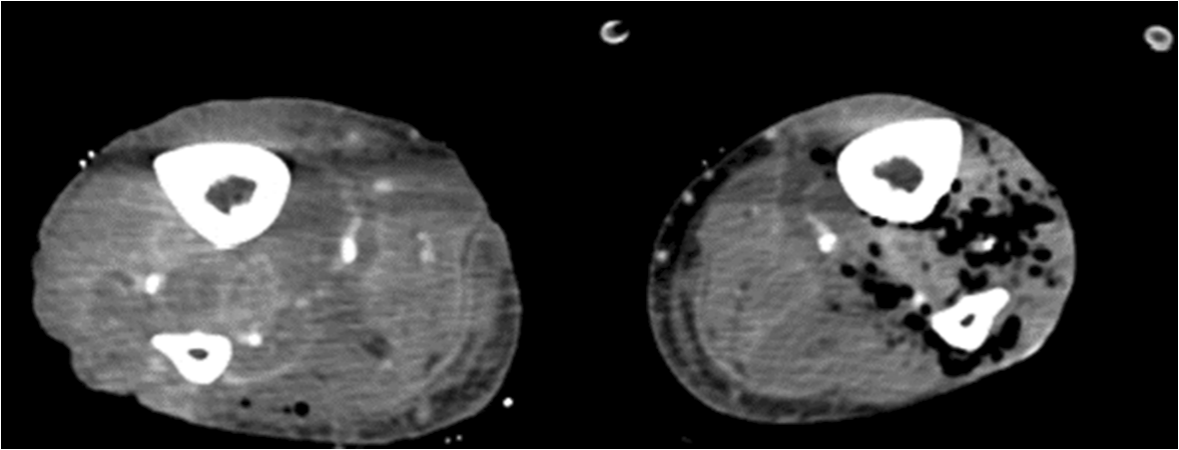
Computed tomography scans of legs demonstrating gas gangrene.
